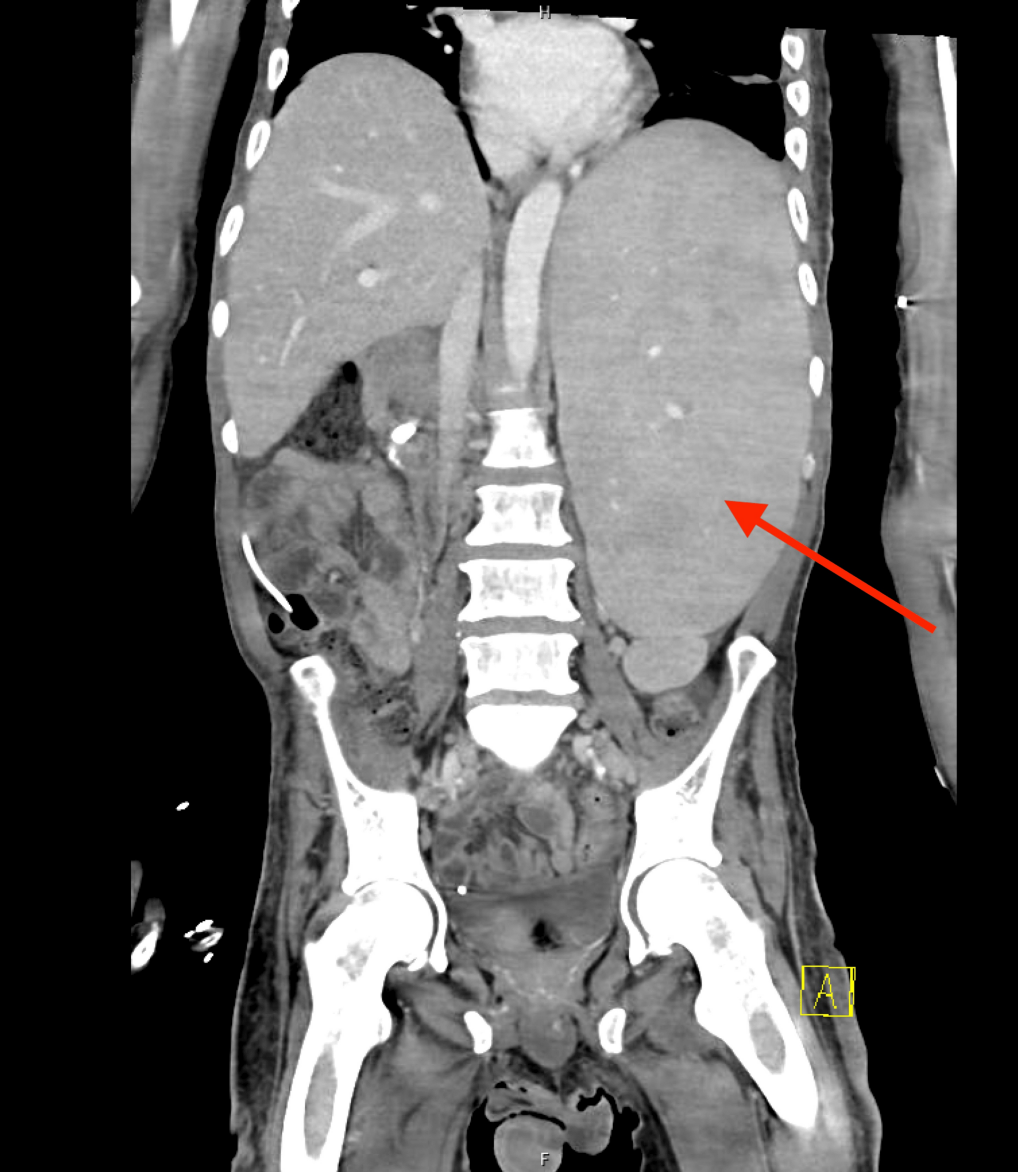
CT scan of the patient’s abdomen and pelvis demonstrating significant splenomegaly
